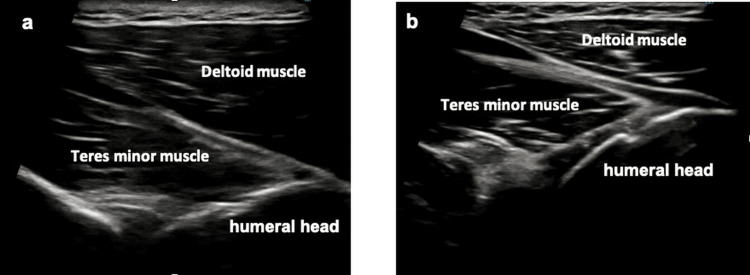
Dynamic ultrasound (US) assessment of the teres minor. a) affected side, b) unaffected side.
